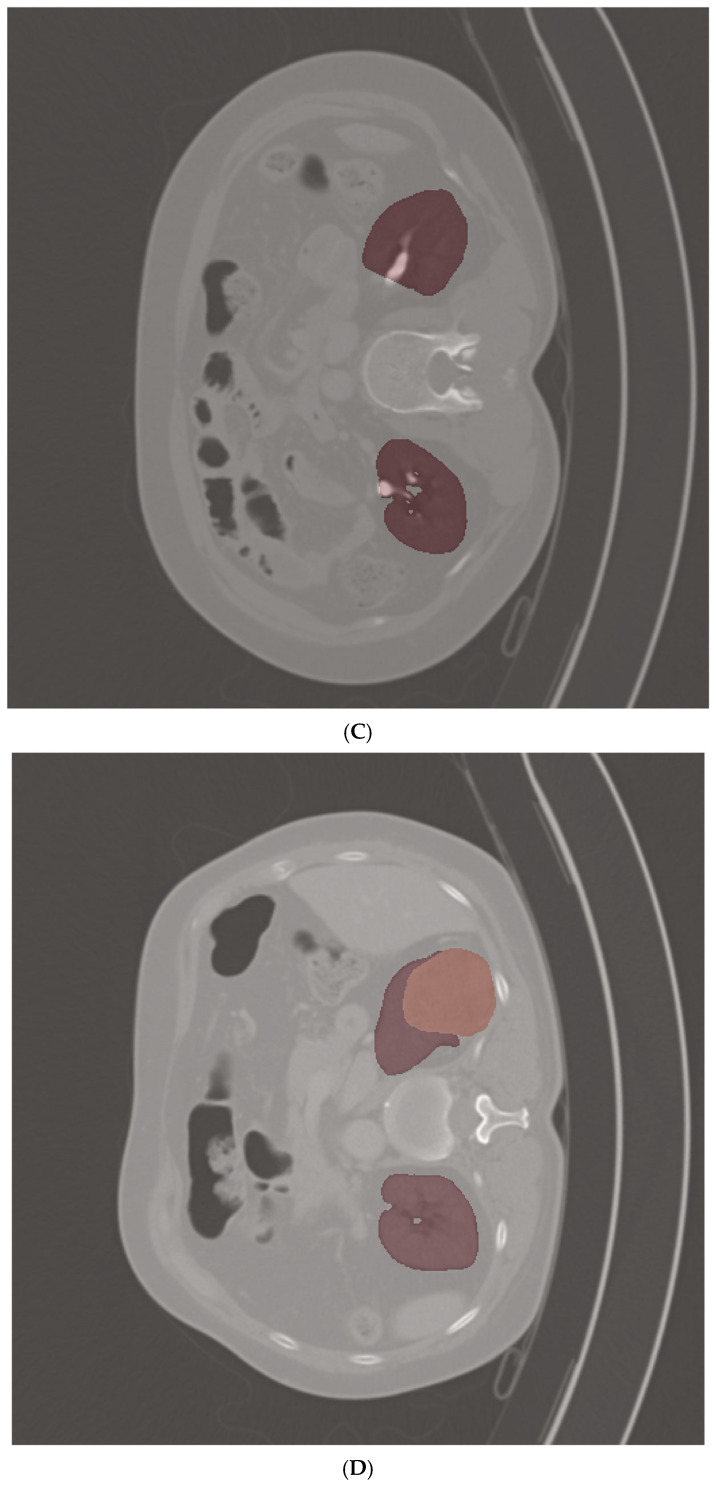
Region of interest (ROI) masks for radiomic feature extraction. (A) Non-contrast phase CT scans overlaid with kidney ROI mask. (B) Arterial phase CT scans overlaid with kidney ROI mask. (C) Delayed phase CT scans overlaid with kidney ROI mask. (D) Portal phase CT scans overlaid with kidney ROI mask.
